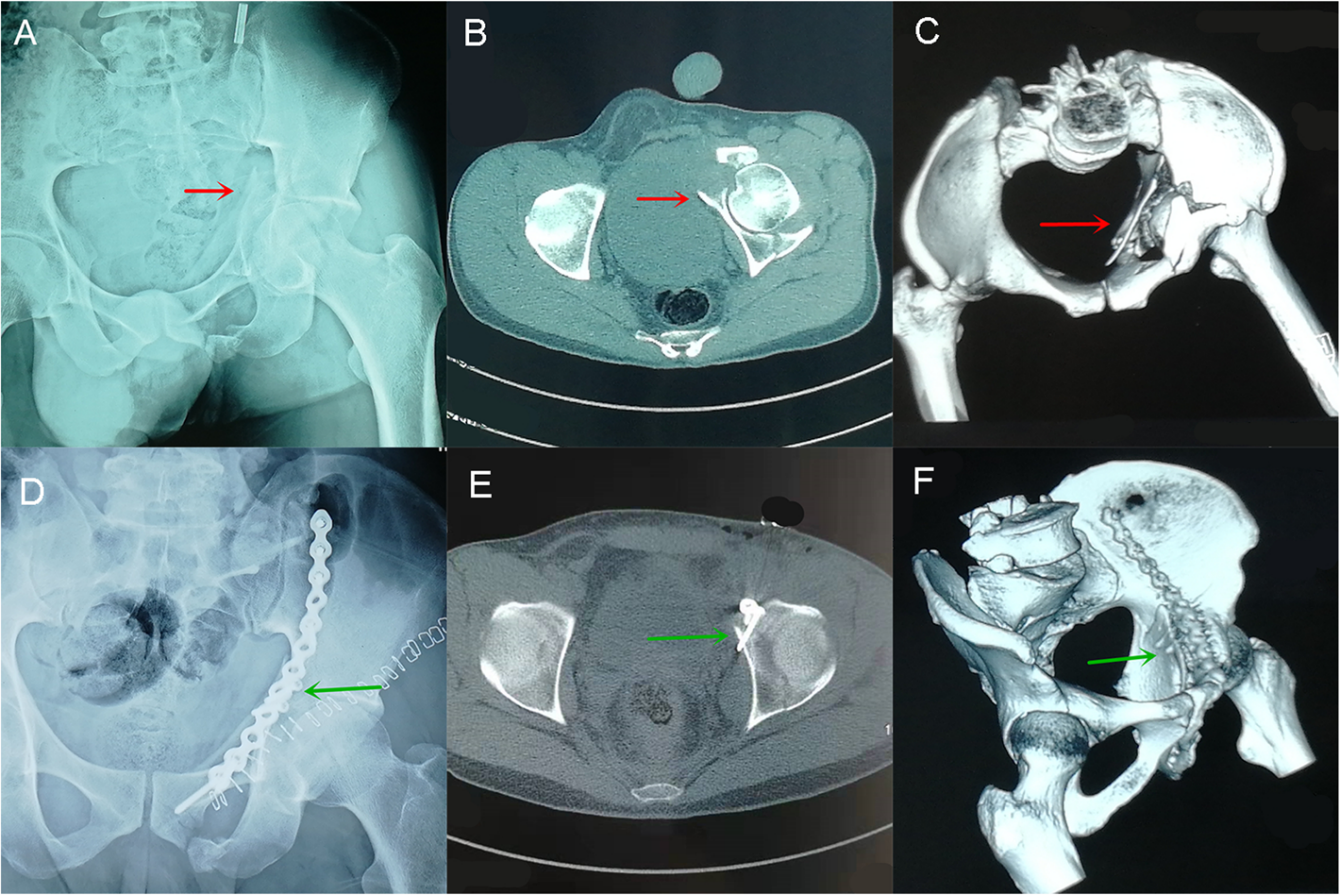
A 45-year-old man was injured by a car accident. a-c: preoperative X-Ray, CT cross section and 3D reconstruction. The red arrow represents the displaced quadrilateral plate fracture. d-f: postoperative X-Ray, CT cross section and 3D reconstruction. Green arrow shows two 3.5-mm cortical bone screws used to fix the quadrilateral plate
